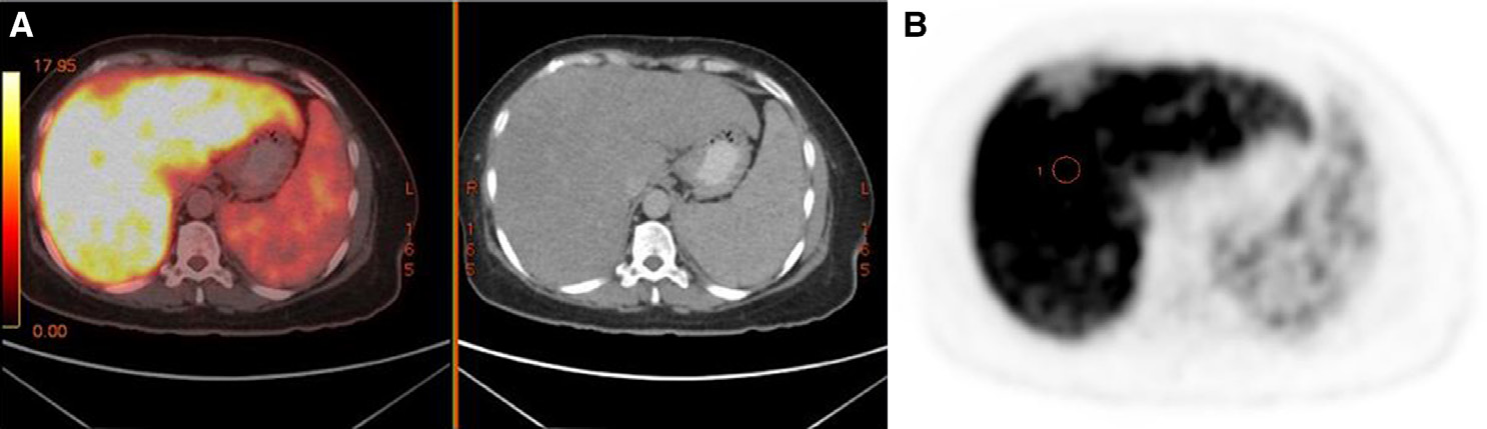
(a) Axial fused PET/CT image and CT image (obtained one hour after administration of 5.2 mCi of ^18^F-FDG) show intense uptake in the liver. (b) Axial PET image shows intense uptake in the liver. FDG, fluorodeoxyglucose; PET, positron emission tomography.
